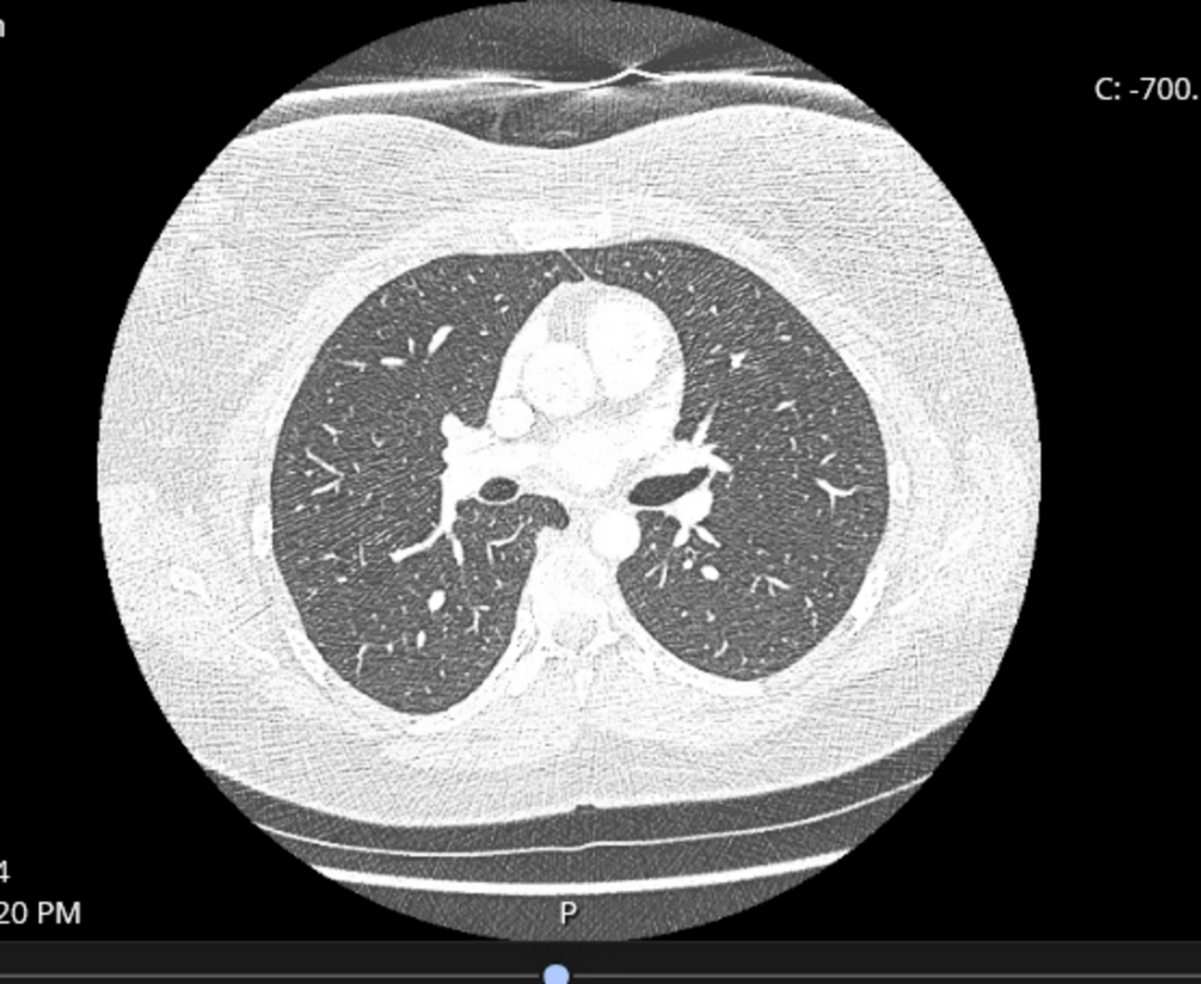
CTA of the chest CTA did not reveal pulmonary embolism CTA: computed tomography angiography
